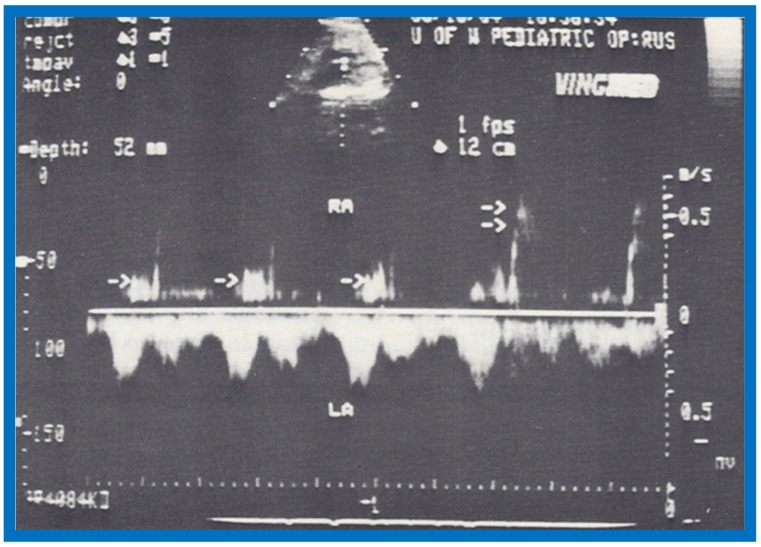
Pulse Doppler recording with the sample volume placed on the left atrial side of the foramen ovale (insert at the top) demonstrating transatrial flow. The majority of the flow is from the right atrium (RA) to the left atrium (LA) (bottom of the baseline). Left-to-right atrial shunt (above the baseline) is also seen, as marked by single arrows in the first three beats. Double arrows on the fourth beat shows Doppler recording of the ascending aortic flow, which serves to reference the timing of ventricle systole. Reproduced from Wilson AD, Rao P.S., Aeschlimann S. [19].
